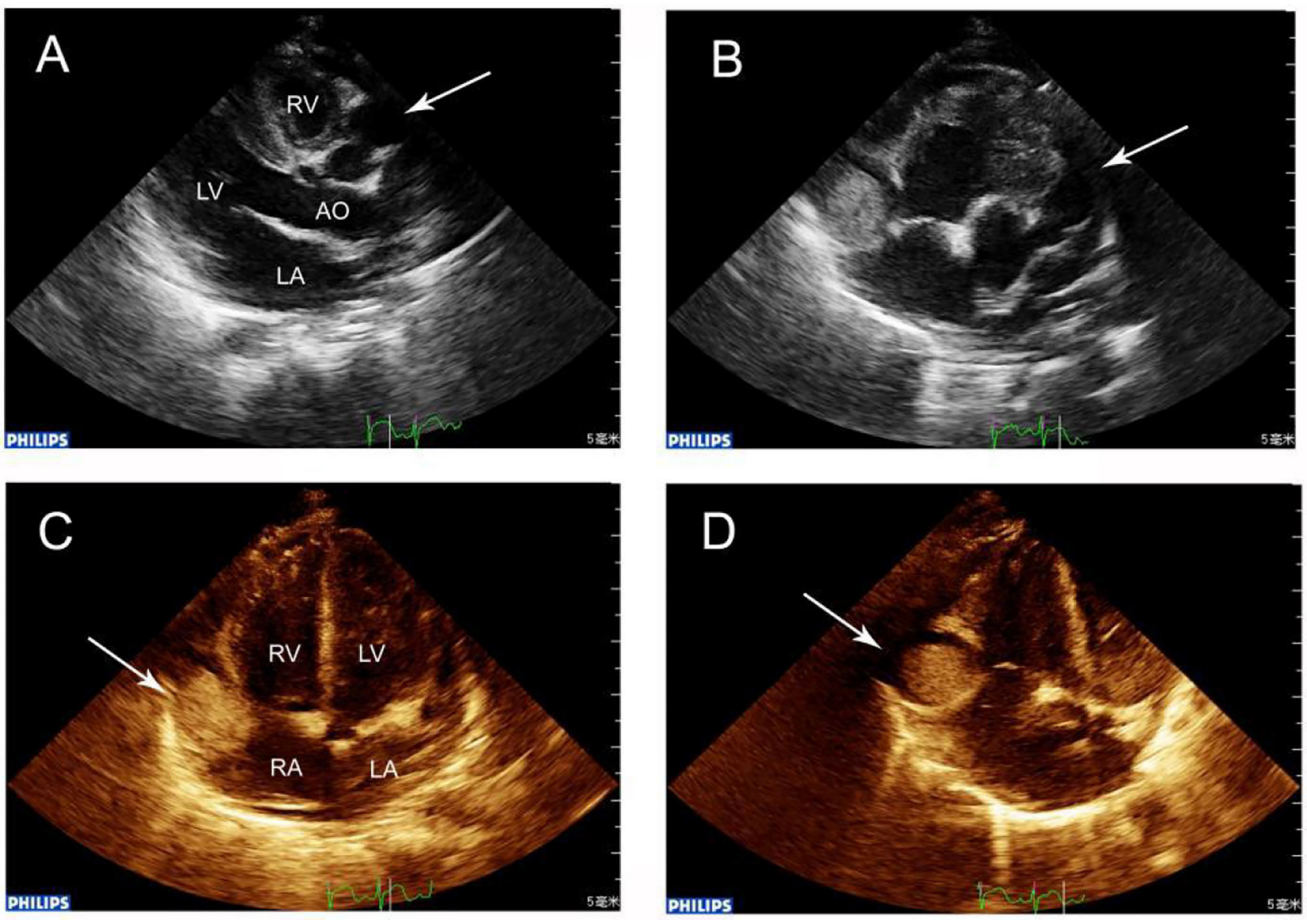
(A) Echocardiography showing RCA dilation (arrow) in the transthoracic long-axis view, showing the characteristic “string-of-beads” pattern. (B) Parasternal shor*t-*axis view showing a giant aneurysm (maximal diameter, 15.0 mm) in the RCA where within 5 mm, a thrombus was located. The LCA was also grossly dilated (arrow). (C) Apex four-chamber view revealing RCA that at the right atrioventricular ring had a giant aneurysm containing a thrombus (arrow). (D) In the giant aneurysm, a 13.0 × 13.0 mm thrombus was observed in echocardiography (arrow).
